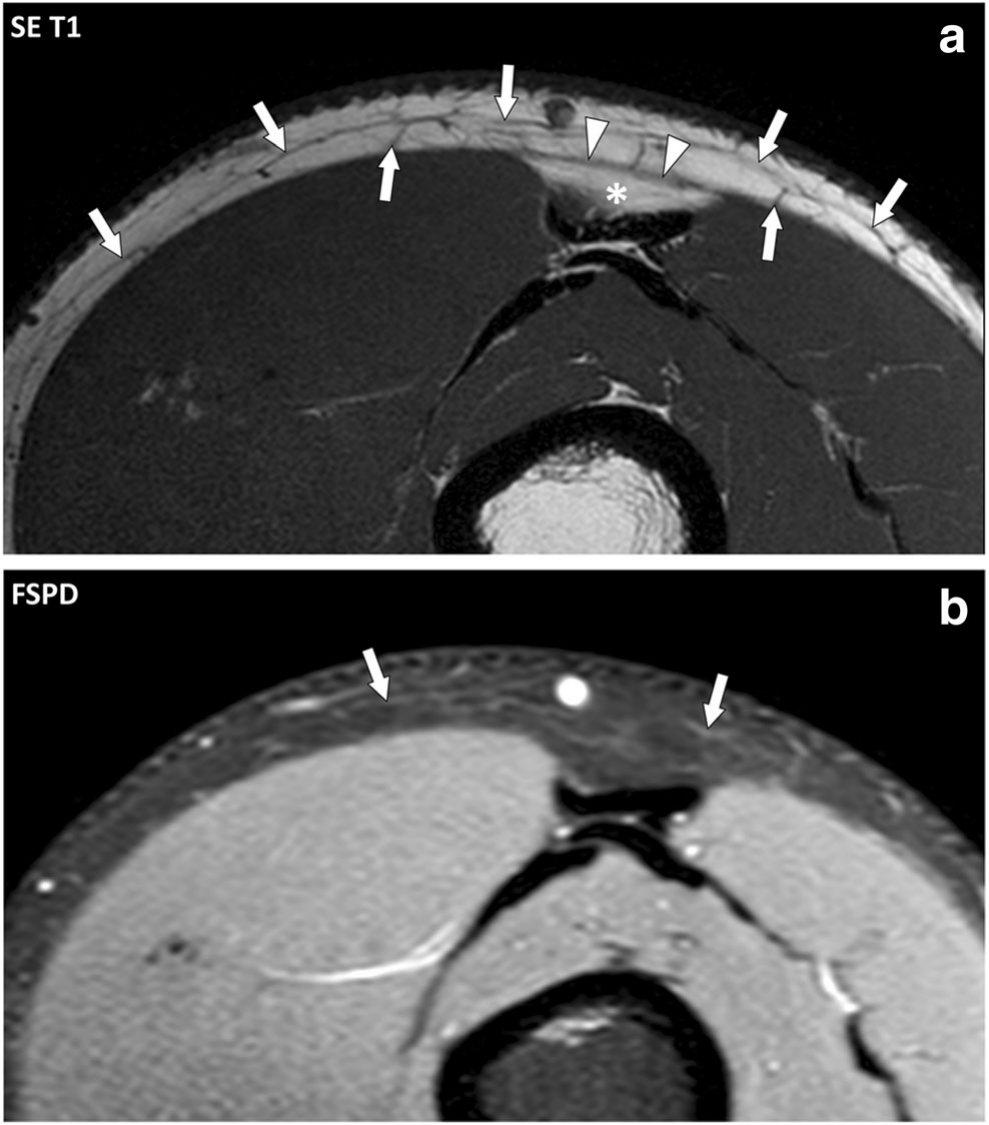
Transverse (a) spin echo (SE) T1 and (b) fat-suppressed proton density (FSPD)-weighted images of the anterior part of the thigh of a 33-year-old normal subject. On the SE T1-weighted image, the fascia superficialis (arrows) appears as a thin reticular network of low signal intensity embedded in the hypodermic fat. The deep peripheral fascia (arrowheads) is only depicted in areas where fat is present deep to it (asterisk). On the corresponding FSPD-weighted image, the fascial system shows low signal intensity and is almost undistinguishable from the adjacent fat
